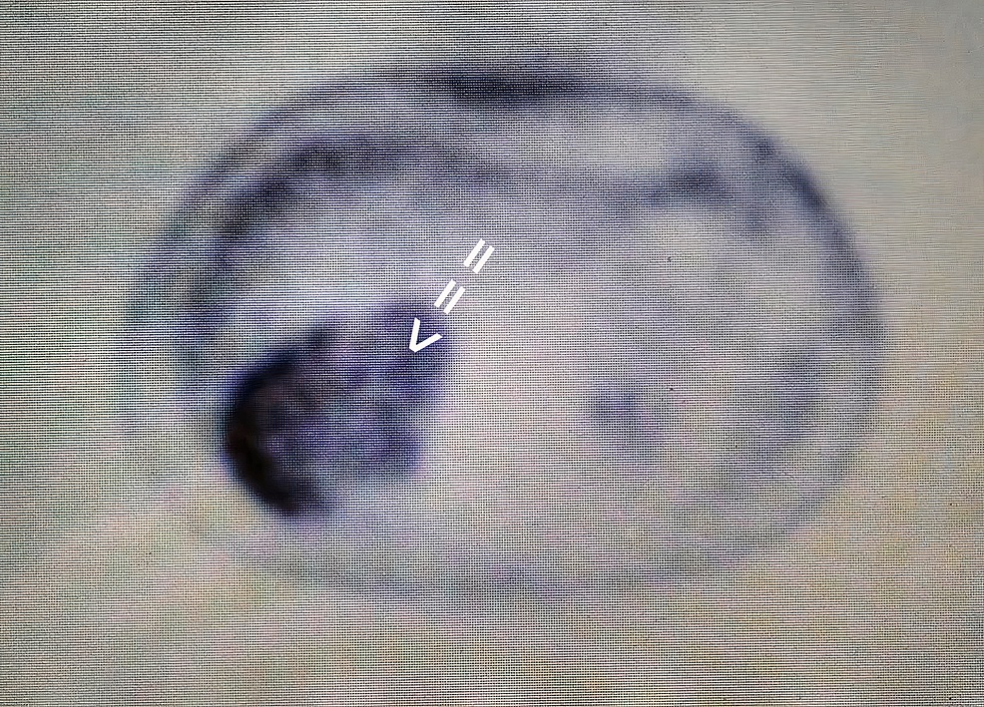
Intense uptake within the right adrenal nodule in fluorodeoxyglucose positron emission tomography (FDG-PET)
